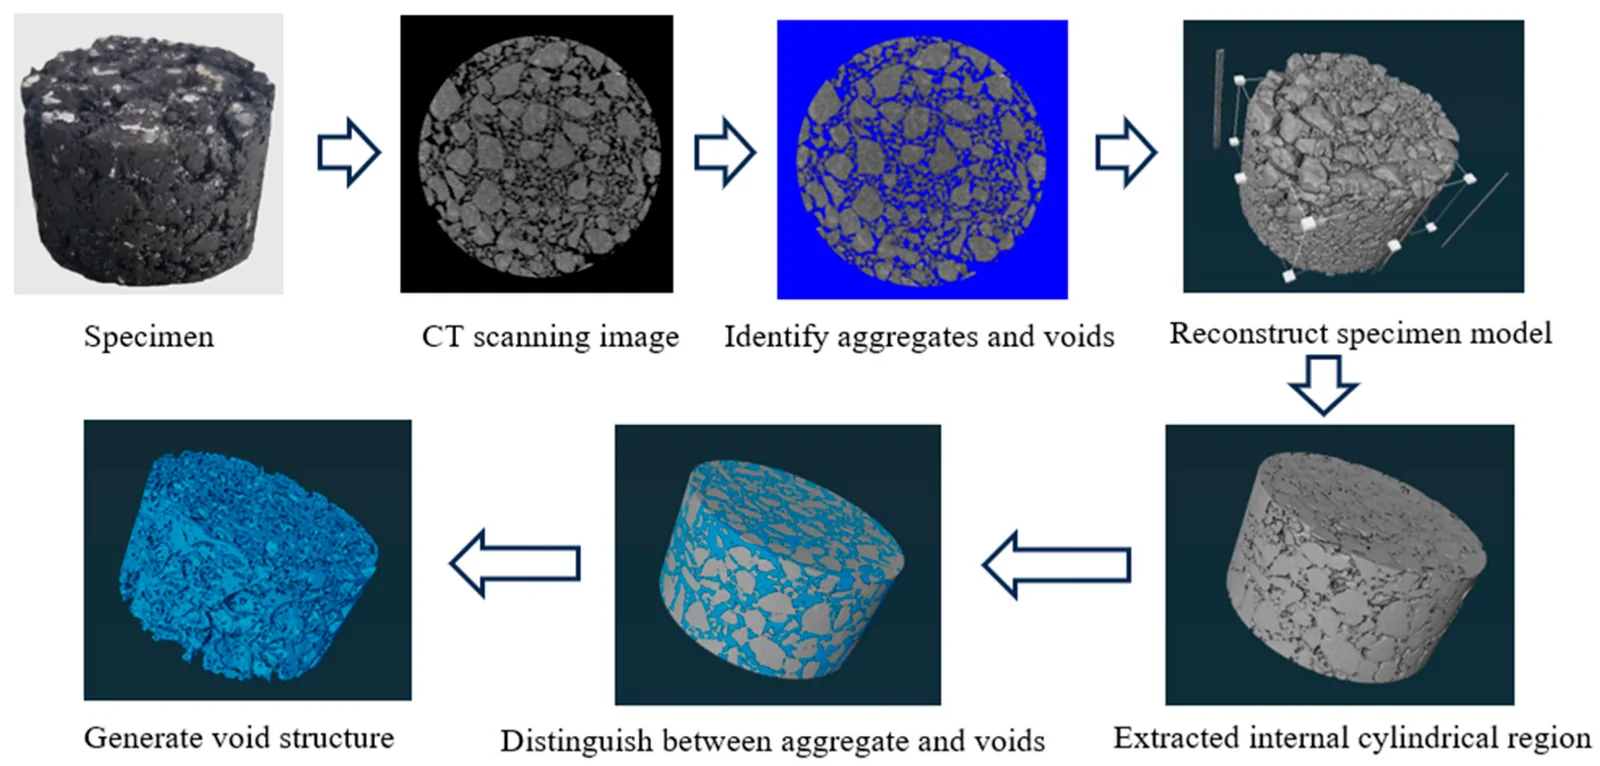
CT scanning three-dimensional reconstruction method.
